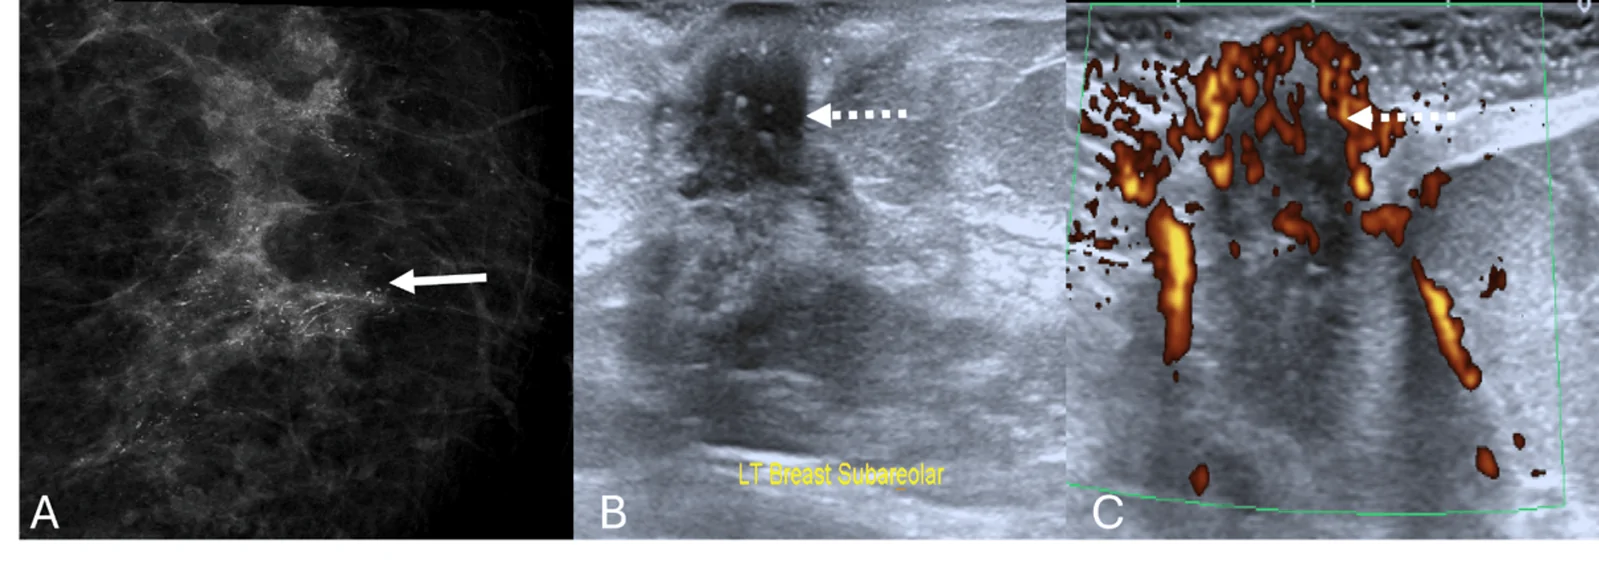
Mammographic and ultrasonographic findings demonstrating pleomorphic calcifications and abnormal nipple-areola complex involvement (A) Spot magnification mammogram of the left breast demonstrating fine linear pleomorphic calcifications in segmental and regional distribution (solid arrow). (B) Ultrasonogram depicting a hetero-echoic left nipple-areolar complex with calcifications and increased vascularity (dotted arrow)
